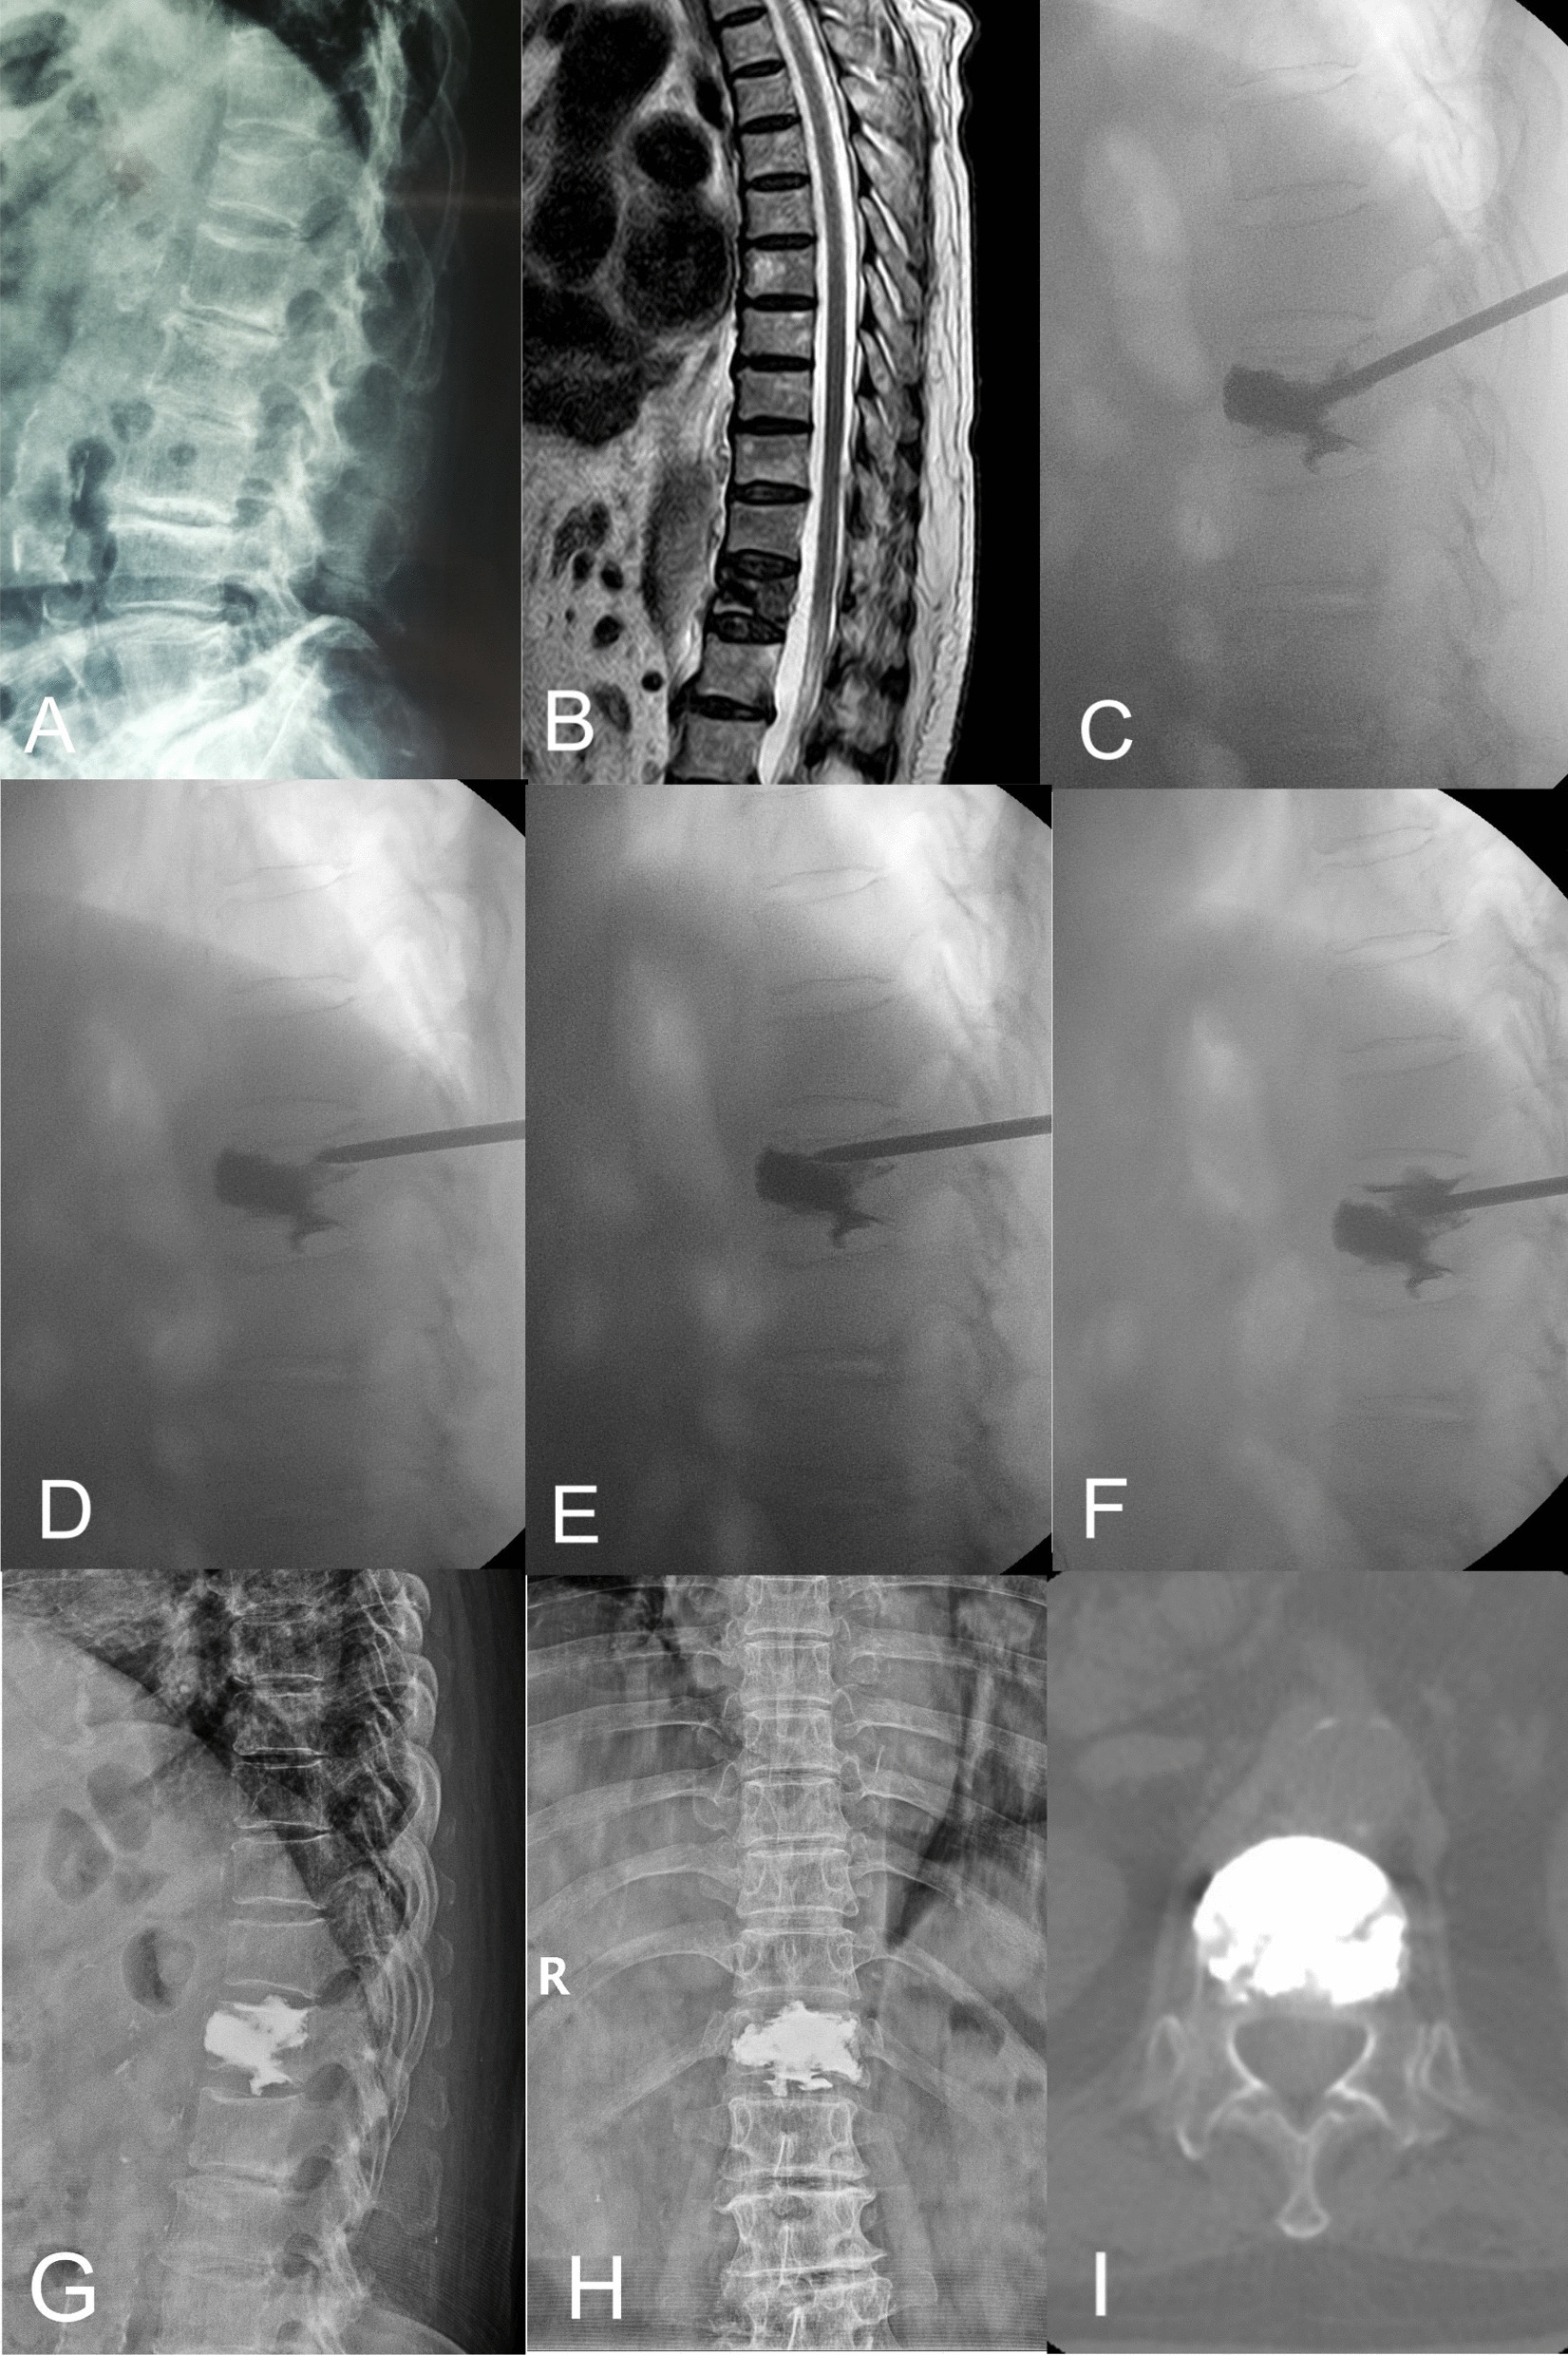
A 71-year-old female patient diagnosis as T12 OVCF was treated with unilateral PKP. A, B Preoperative X-ray and thoracic MRI showed fresh compressive fracture at T12. C Intraoperative fluoroscopy showed insufficient bone cement distribution at the upper part with cement leakage in the lower disc space. D, E Working channel was retracted to the posterior wall of the vertebra, and put the working channel to the area lack of cement distribution. F After second injection, sufficient cement distribution was obtained. G–I Postoperative X-ray and CT showed sufficient bone cement in T12 vertebral body
